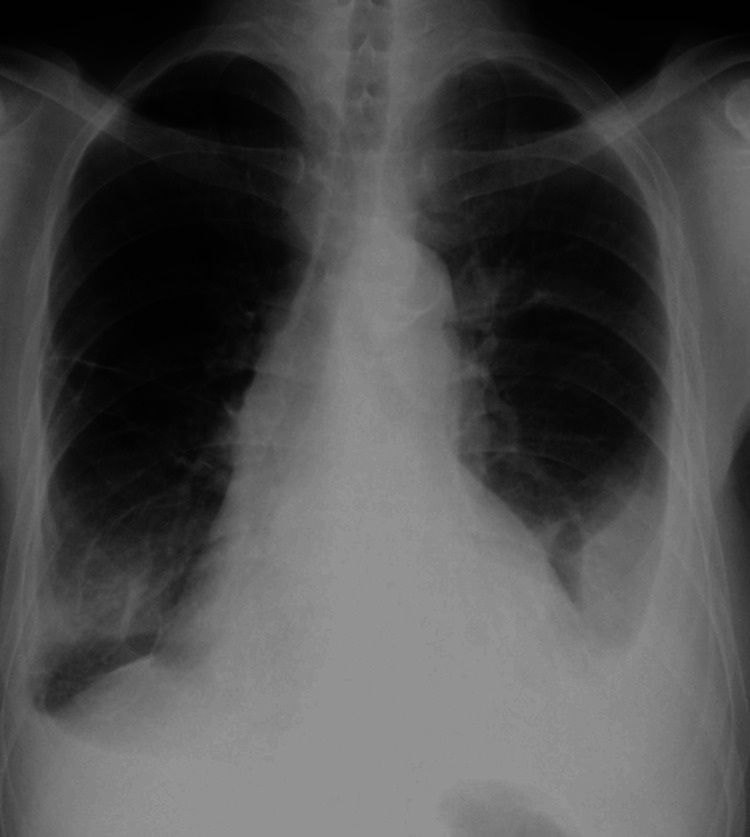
Chest x-ray seven months after hospital discharge
